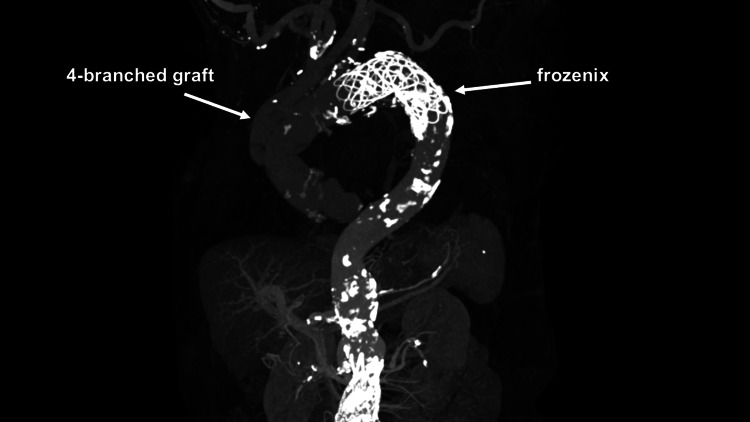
Postoperative computed tomography angiography Postoperative computed tomography angiography revealing excluded arch aneurysm
